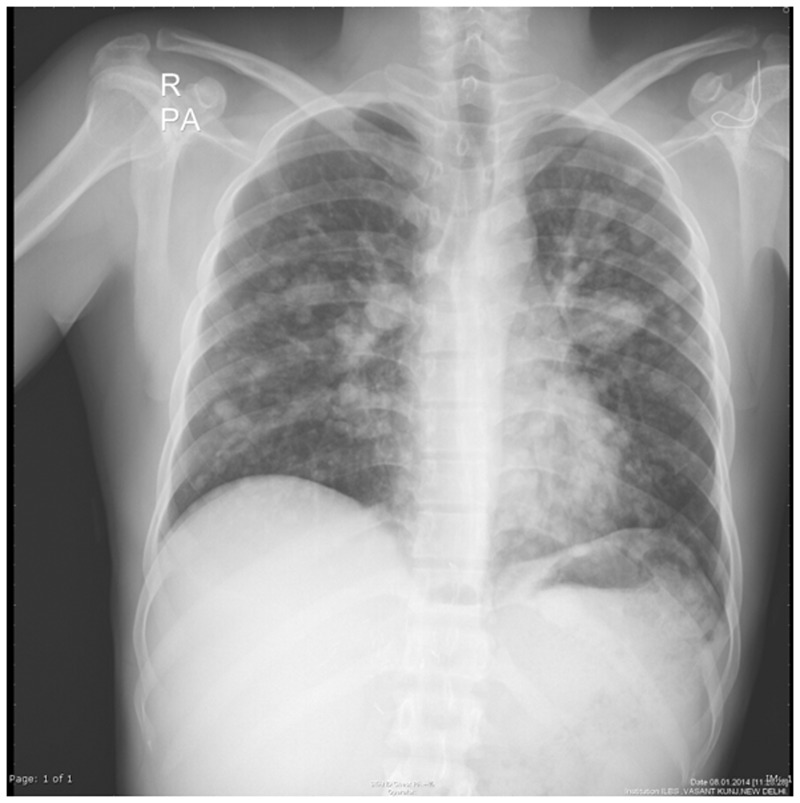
Metastases. Chest radiograph in a 50-year-old man with liver cirrhosis and hepatocellular carcinoma showing multiple nodular opacities in bilateral lungs, suggestive of hematogenous metastases.
